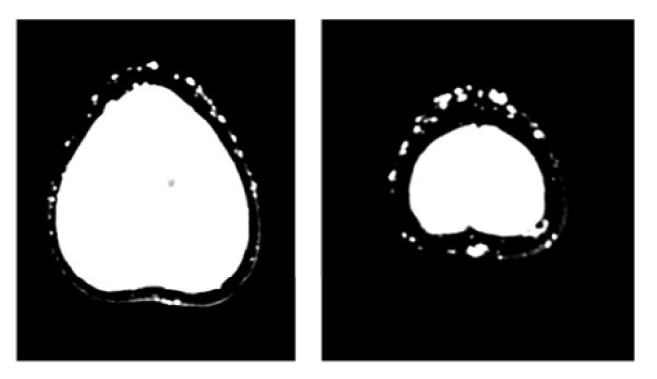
Extracranial subcutaneous ectopic calcifications (CT scan).
